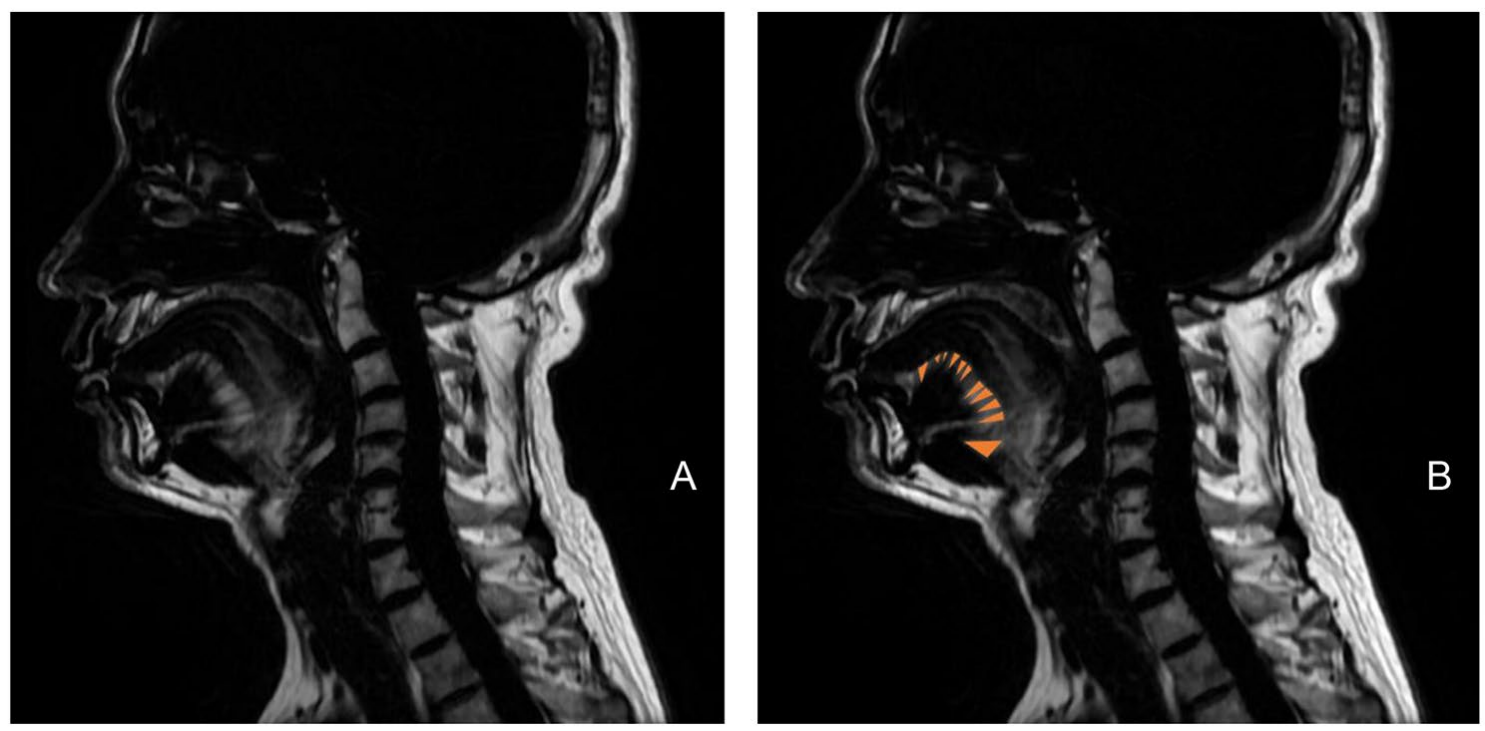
Characteristics of tongue fat. The sagittal slice of MRI in tongue fat which characteristics of distribution are fanned out (A), fat was marked by yellow (B)
